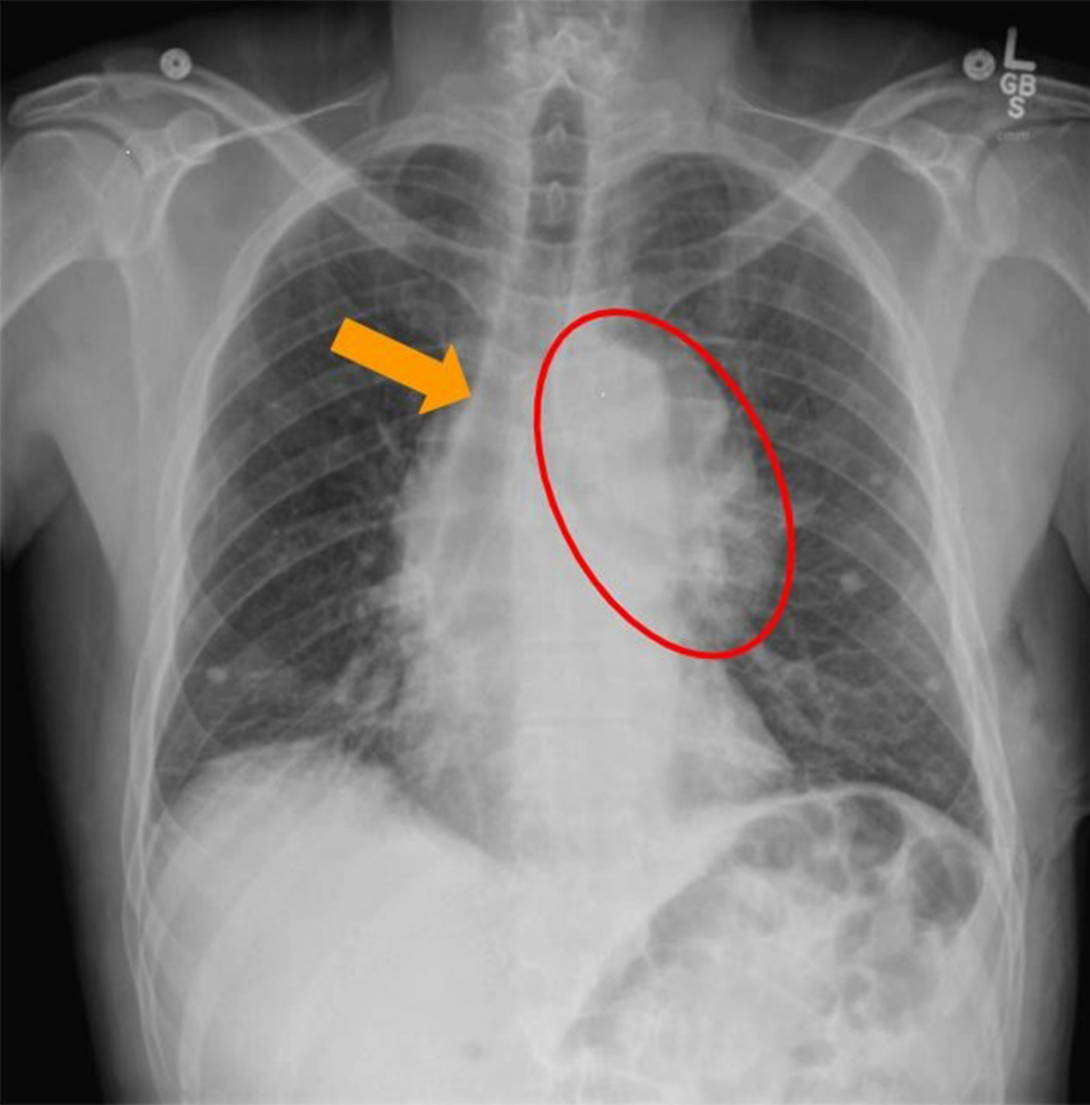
Preoperative chest X-ray. The rightward tracheal shift (orange) due to compression from the left-sided thymoma (red) is shown.
